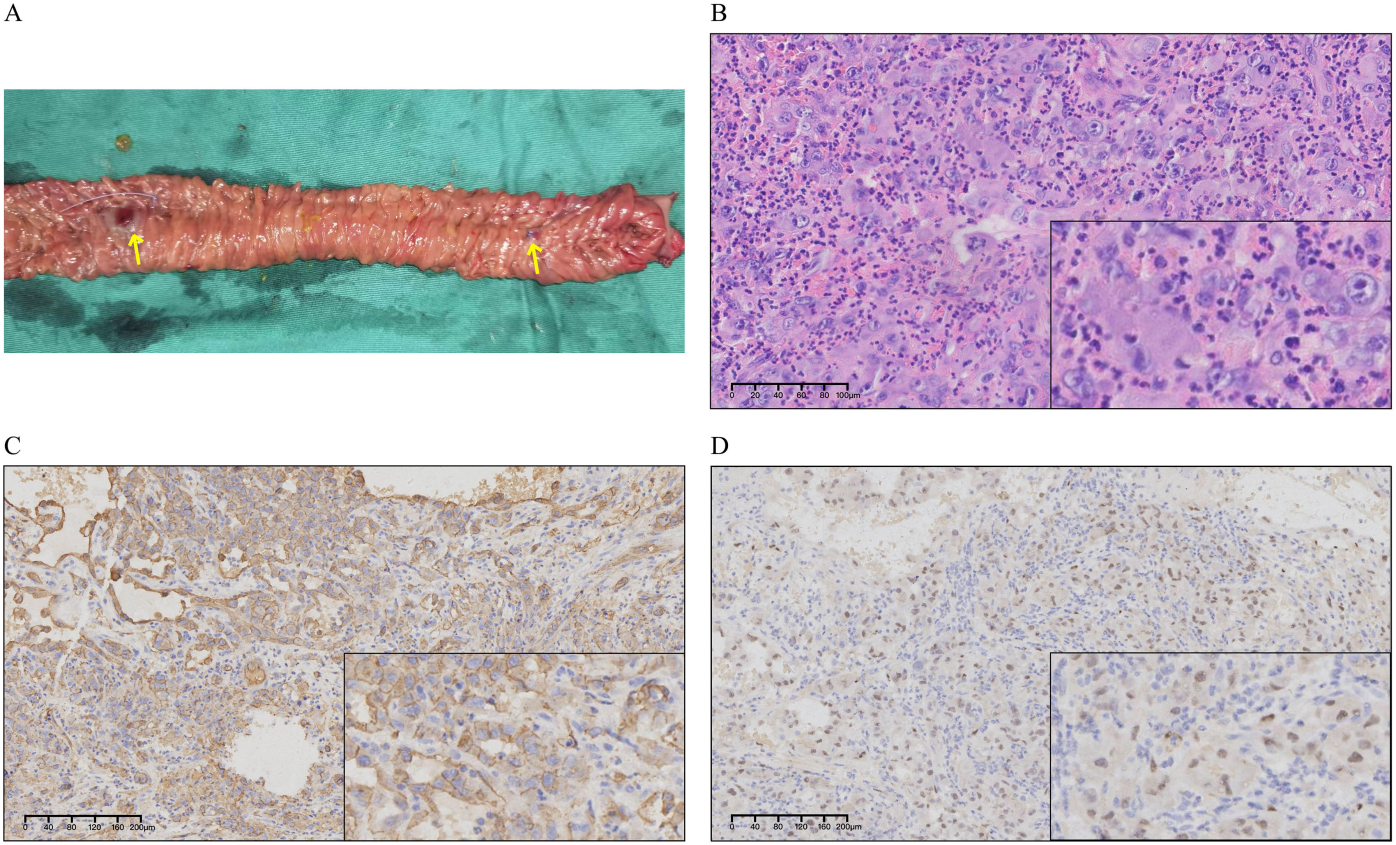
PET-CT after surgery suggesting multiple metastases. (A) One 5-mm nodule with high fluorodeoxyglucose (FDG) of multiple nodules in both lungs. (B) Muscles of the right upper arm with increased FDG. (C) Soft tissue density foci in the upper mediastinum with unevenly increased FDG. (D) Multiple foci of increased FDG in the sacrum with partial bone destruction. All of these lesion foci with elevated FDG were suspicious for tumor metastasis.
